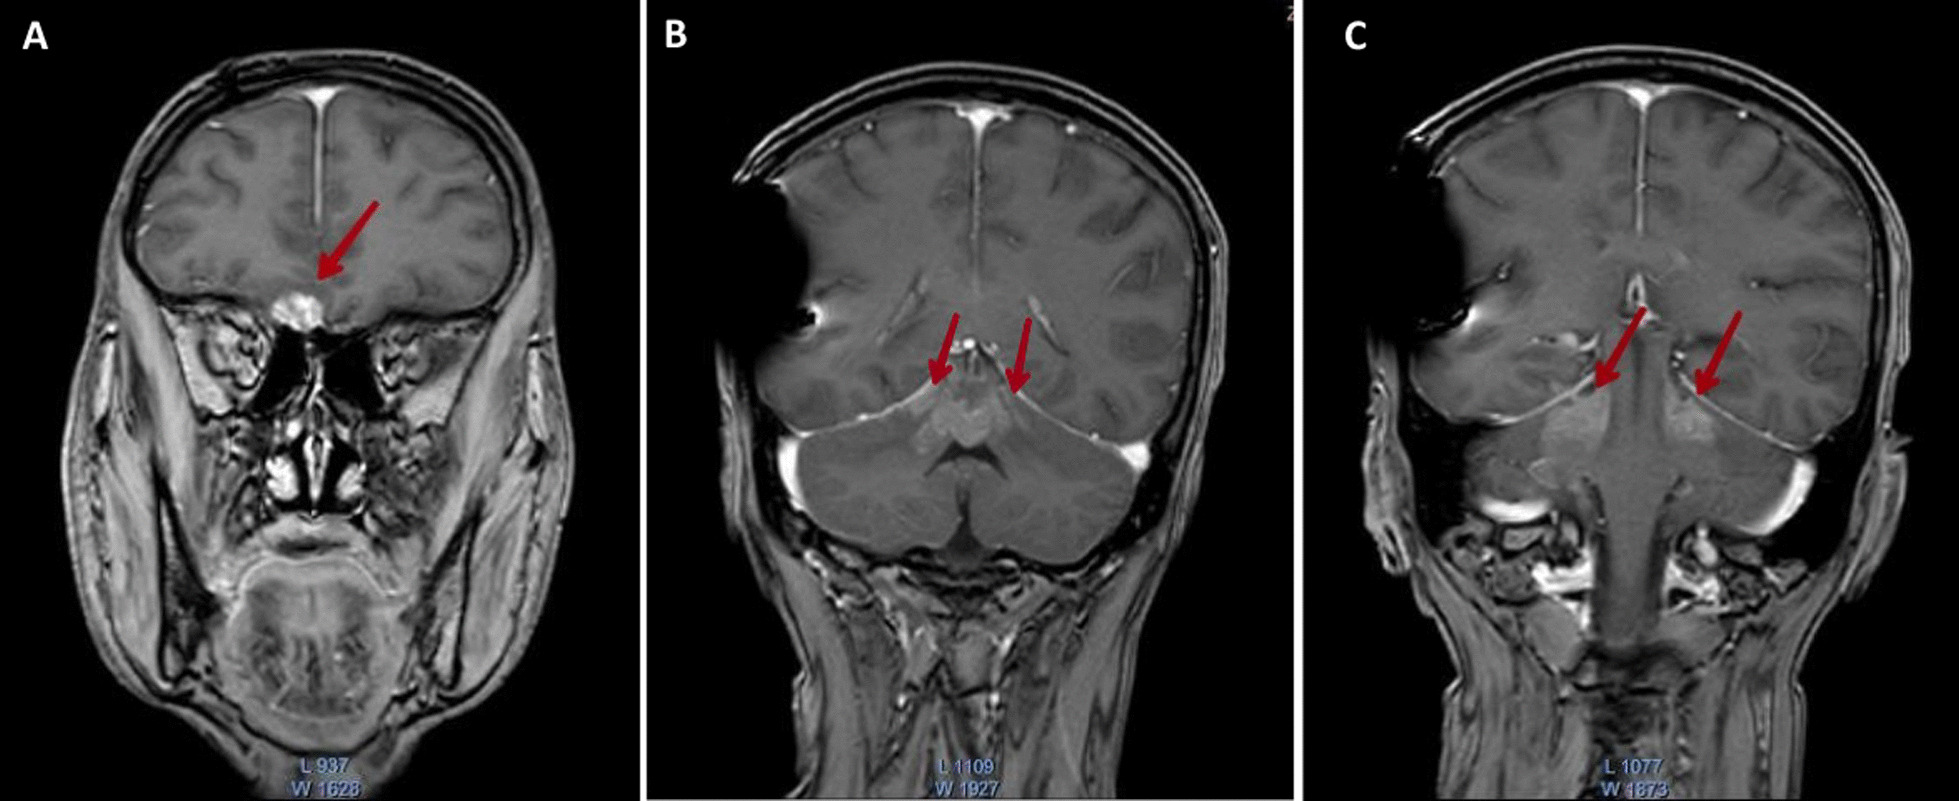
T1-weighted postcontrast MRI images show brain metastases: a one enhancing lesion in the right temporal lobe in transversal view, b lesions around pons Varolii in coronal view, and c lesions around the midbrain in coronal view
